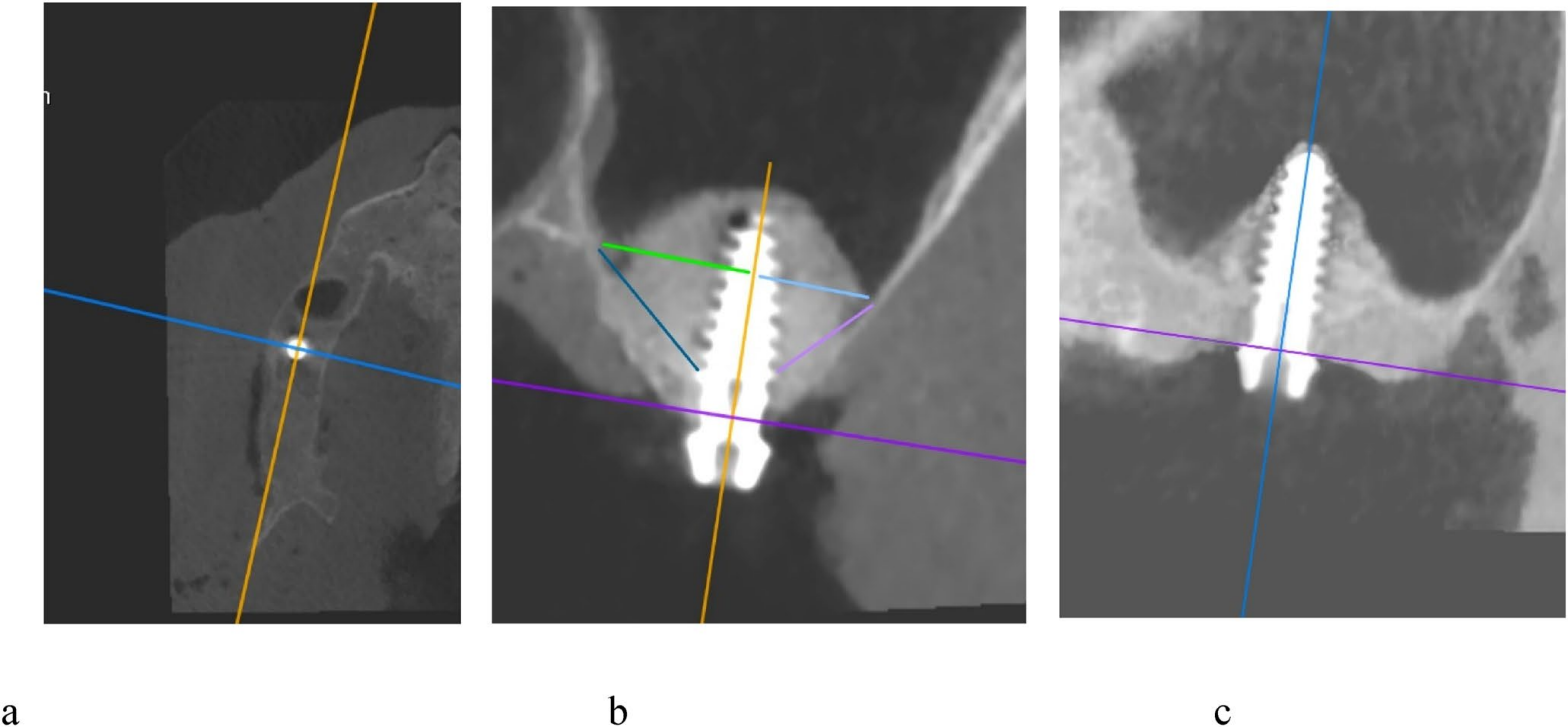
Assessment of membrane detachment. A reference framework was established on the postoperative CBCT to ensure standardization at each site. (a) Mesiodistal (orange line) and buccopalatal (blue) axes were defined in the axial plane. (b) Vertical (orange) and mesiodistal (purple) axes, buccal (light blue) and palatal (green) orthogonal projections were defined in the coronal plane, and detachment occurred on buccal (light purple) and palatal (dark blue) surfaces. (c) Mesial–distal detachments were measured in the mesiodistal axis (vertical axis in blue and mesiodistal axis in purple).
